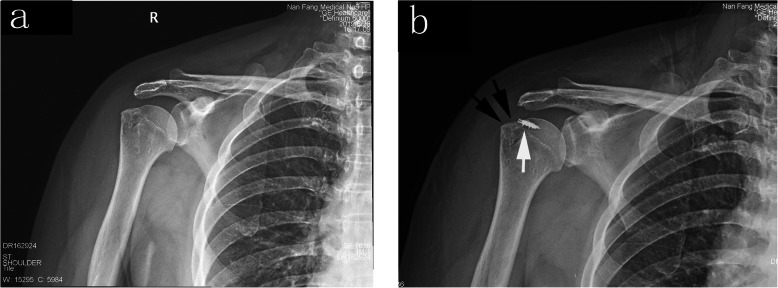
Anterior view of the radiograph. The anchor was inserted 5 mm lateral to the articular cartilage at a 45 degree angle (white arrow). Seven to nine bone marrow vents were created in the greater tuberosity (black arrow)
